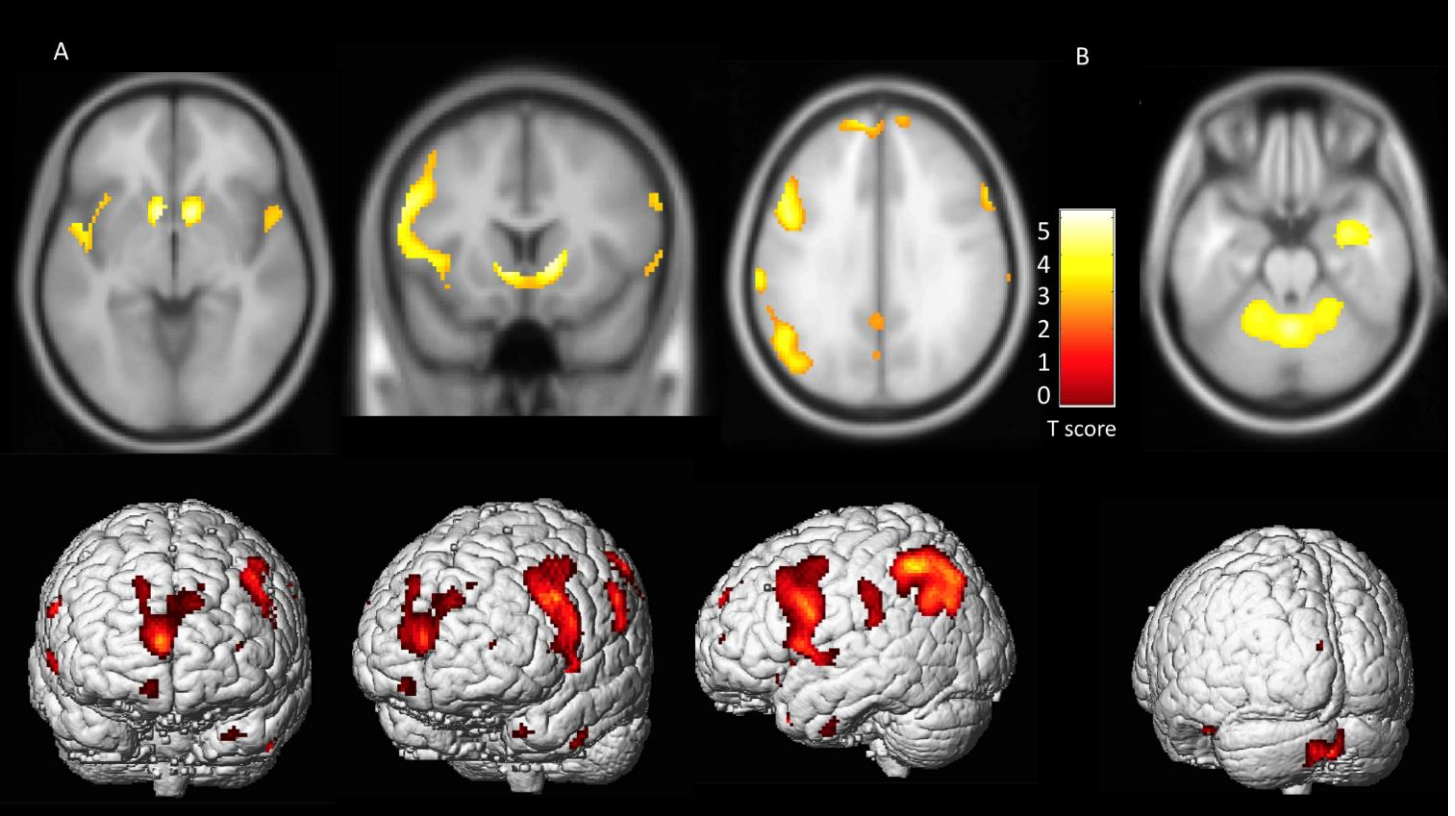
The metabolism of FDG in IPD patients compared to healthy controls. Brain areas with increased/decreased glucose metabolism are superimposed on the Montreal Neurological Institute template (Top row) (*p* < 0.001, uncorrected) and the 3D render (Bottom row). A) Significant hypometabolism in bilateral putamen, caudate, anterior cingulate, parietal lobe and prefrontal cortex was identified. B) The relative hypermetabolism was identified in the cerebellum and vermis.
